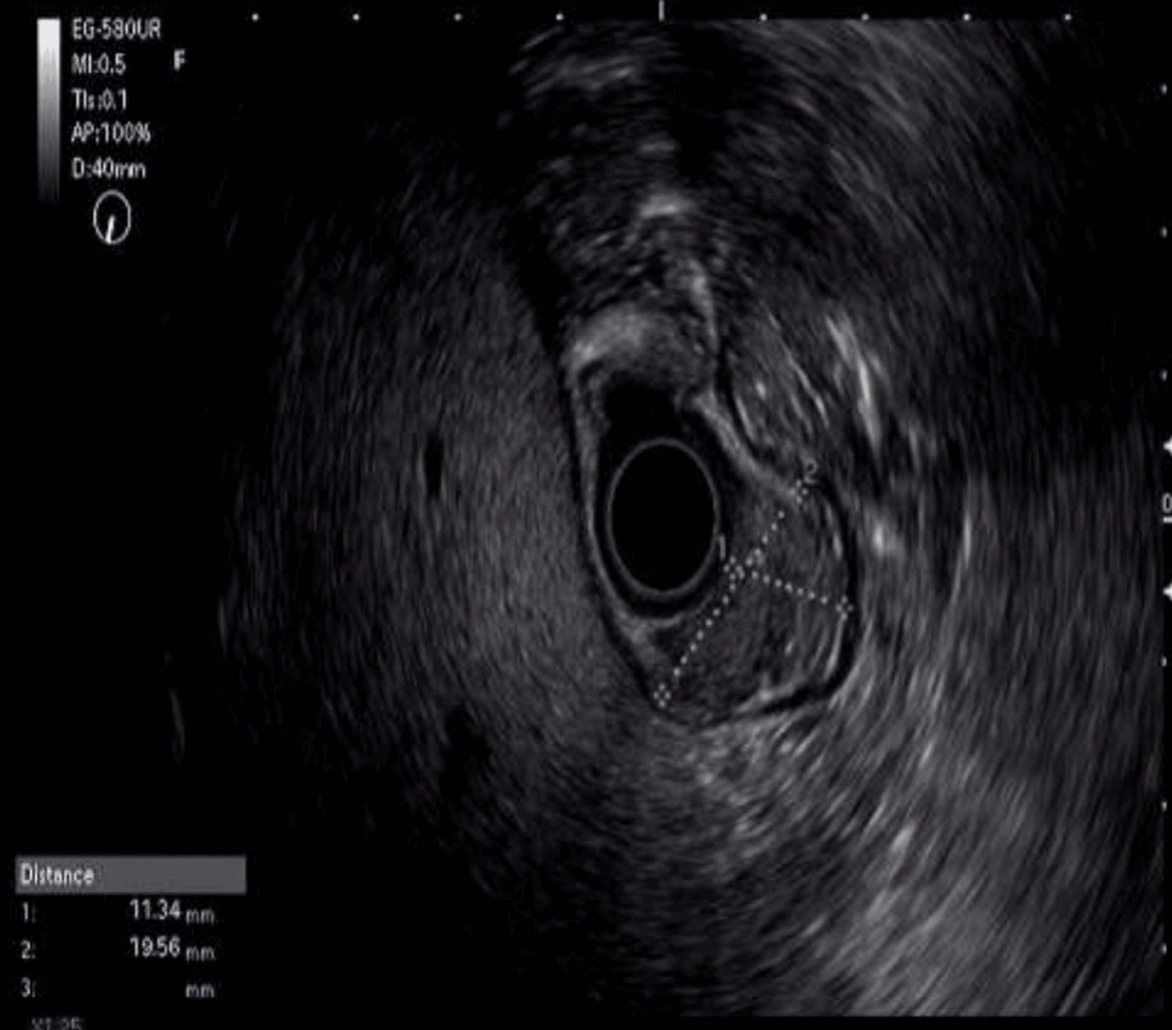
Endoscopic ultrasonography view of gastric glomangioma.
